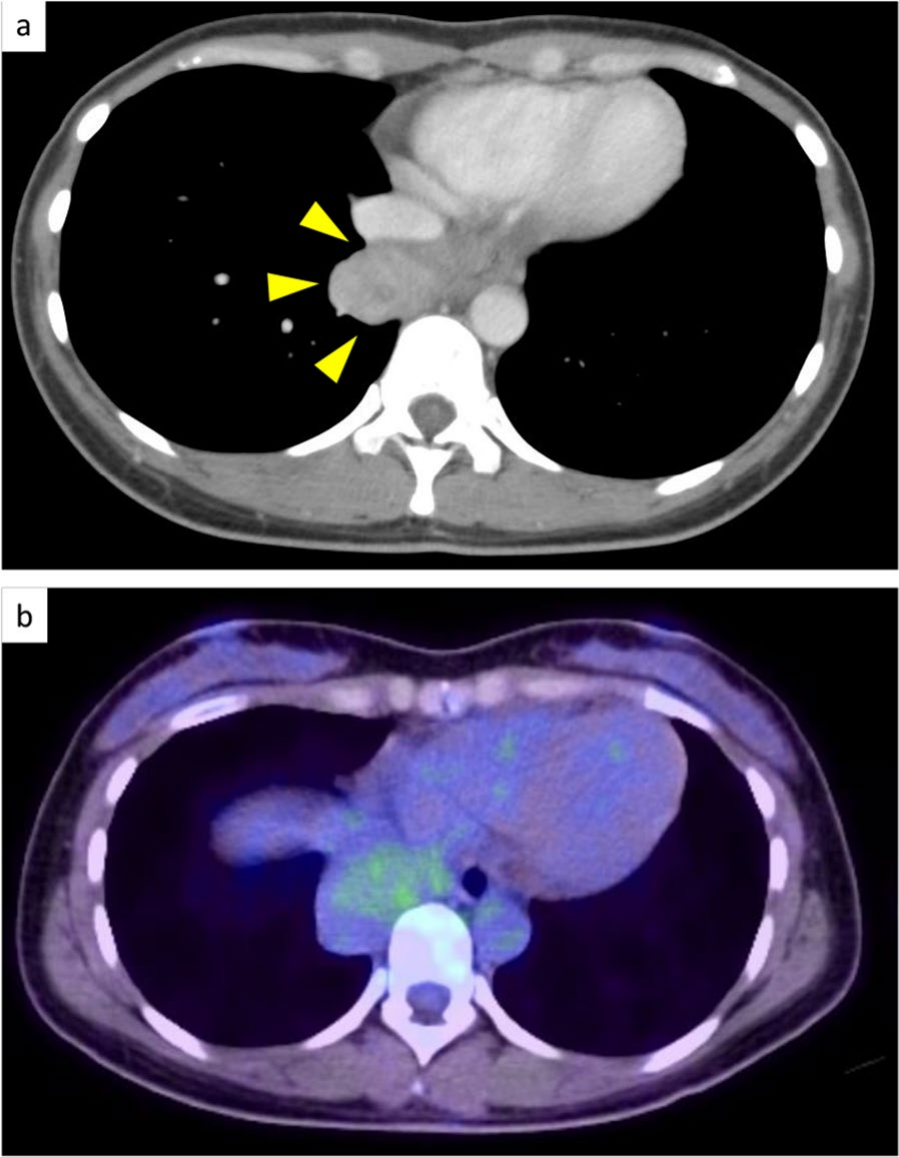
a CT image showing a large mass abutting the inferior vena cava, and esophagus (yellow arrows). b 18F-fluorodeoxyglucose positron emission tomography with CT (FDG-PET/CT) image showing FDG accumulation in the tumor with a maximum standardized uptake (SUVmax) of less than 2.0
